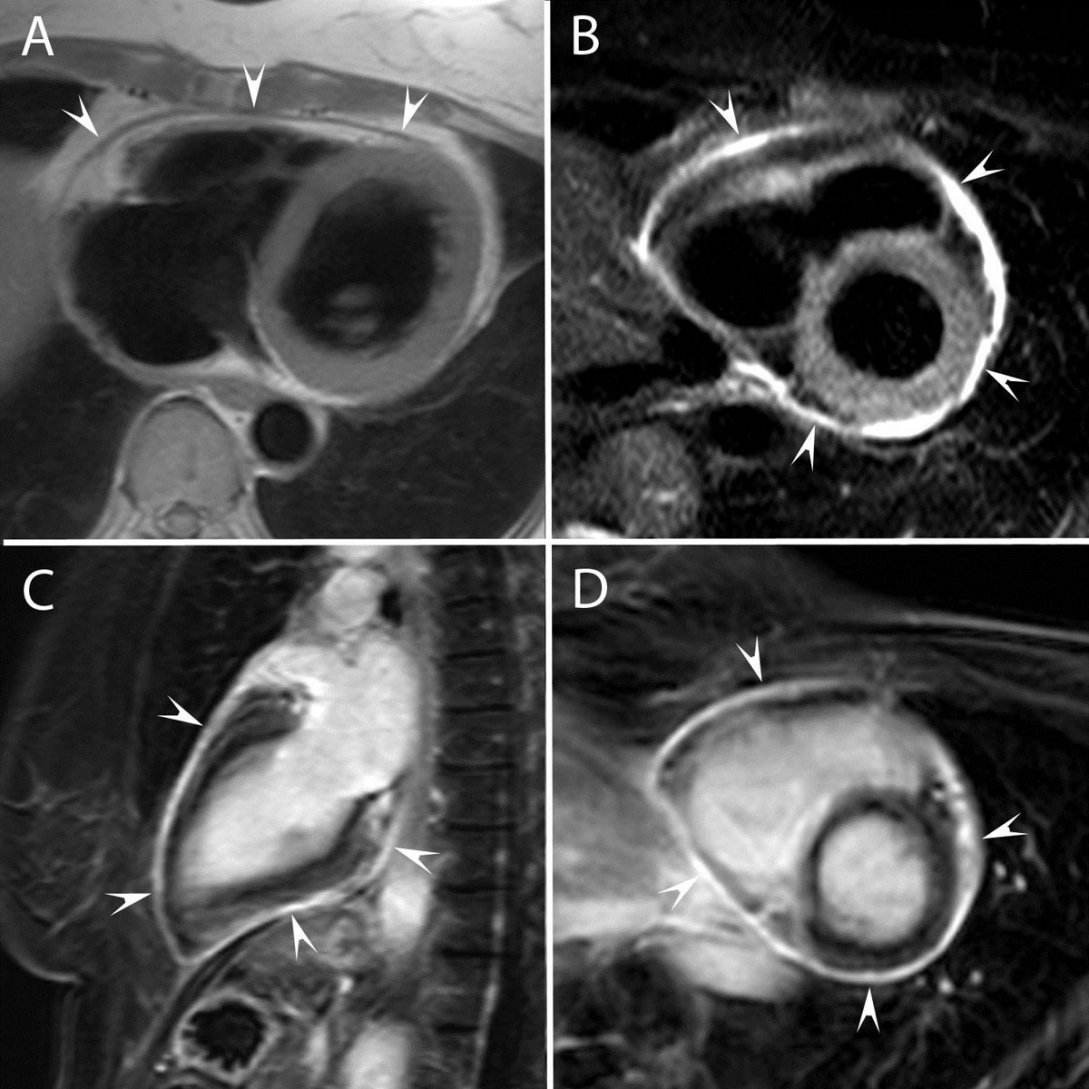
Recent history of acute idiopathic pericarditis, presenting recurrent complaints of chest pain and elevated CRP values. Initial TTE showed minimal pericardial effusion. Axial T1-weighted image (A), short-axis T2-weighted image (B), LGE-image in vertical long-axis (C) and short-axis (D). Minimally thickened pericardial layers (2.5 mm thickness) with SI similar to that of myocardial tissue on T1-weighted imaging (arrowheads, A). High SI on T2-weighted imaging (arrowheads, B), and strong pericardial on LGE-imaging (arrowheads, C,D). No evidence of residual pericardial fluid.
